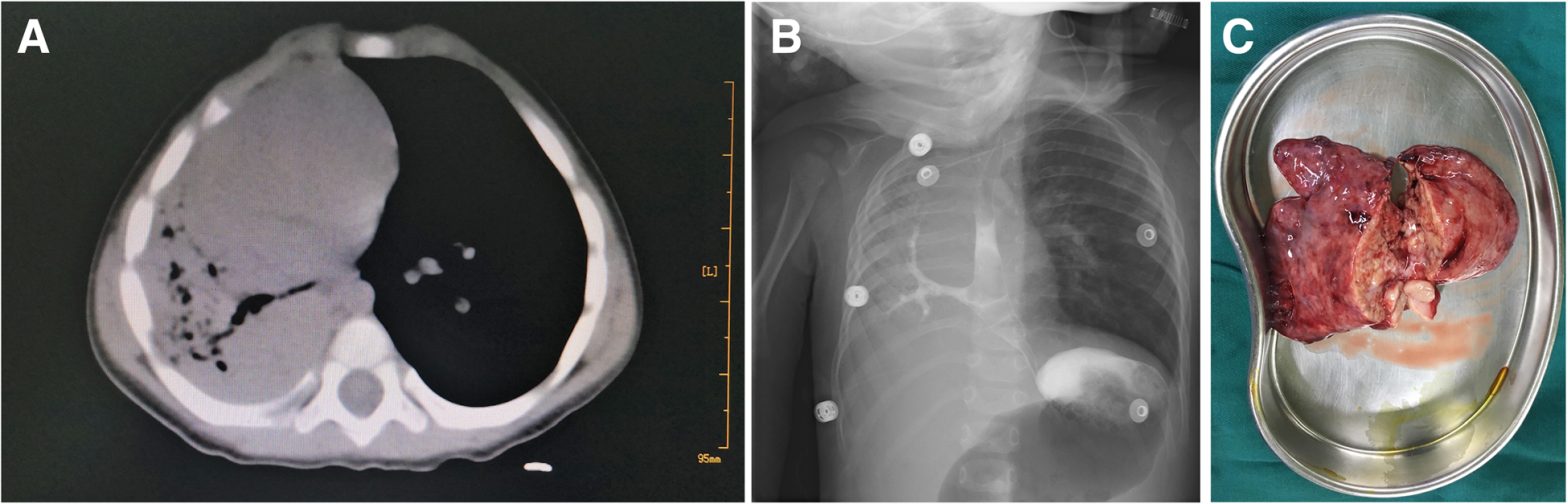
a CT scan showed hypoplasia of the right lung and the right mainstem bronchus originating from the distal esophagus. b The UGI revealed filling of the right main bronchus from the esophagus with opacification of the bronchial tree on the right side. c Diffuse abscesses were formed in the right lung parenchyma
